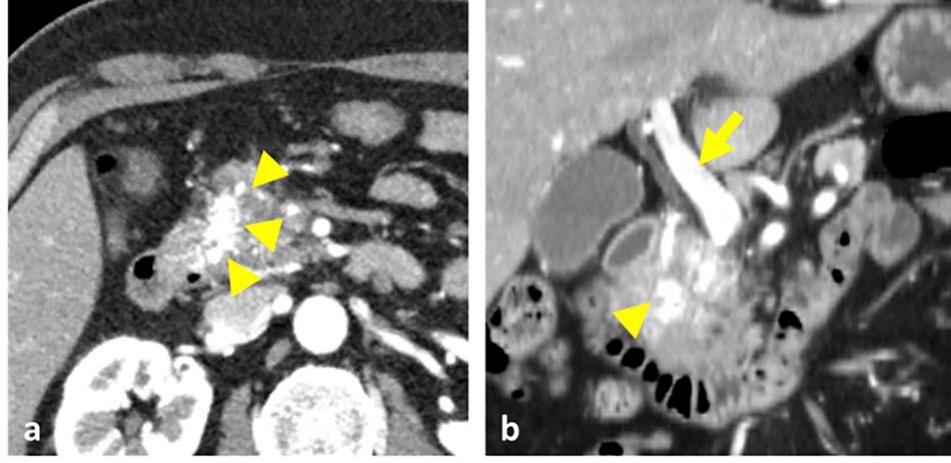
Contrast-enhanced CT images. (a) Vessels in the pancreatic head were strongly enhanced during the arterial phase (arrowheads). (b) Both the vessels in the pancreas head (arrowhead) and the portal vein (arrow) were enhanced at the arterial phase.
